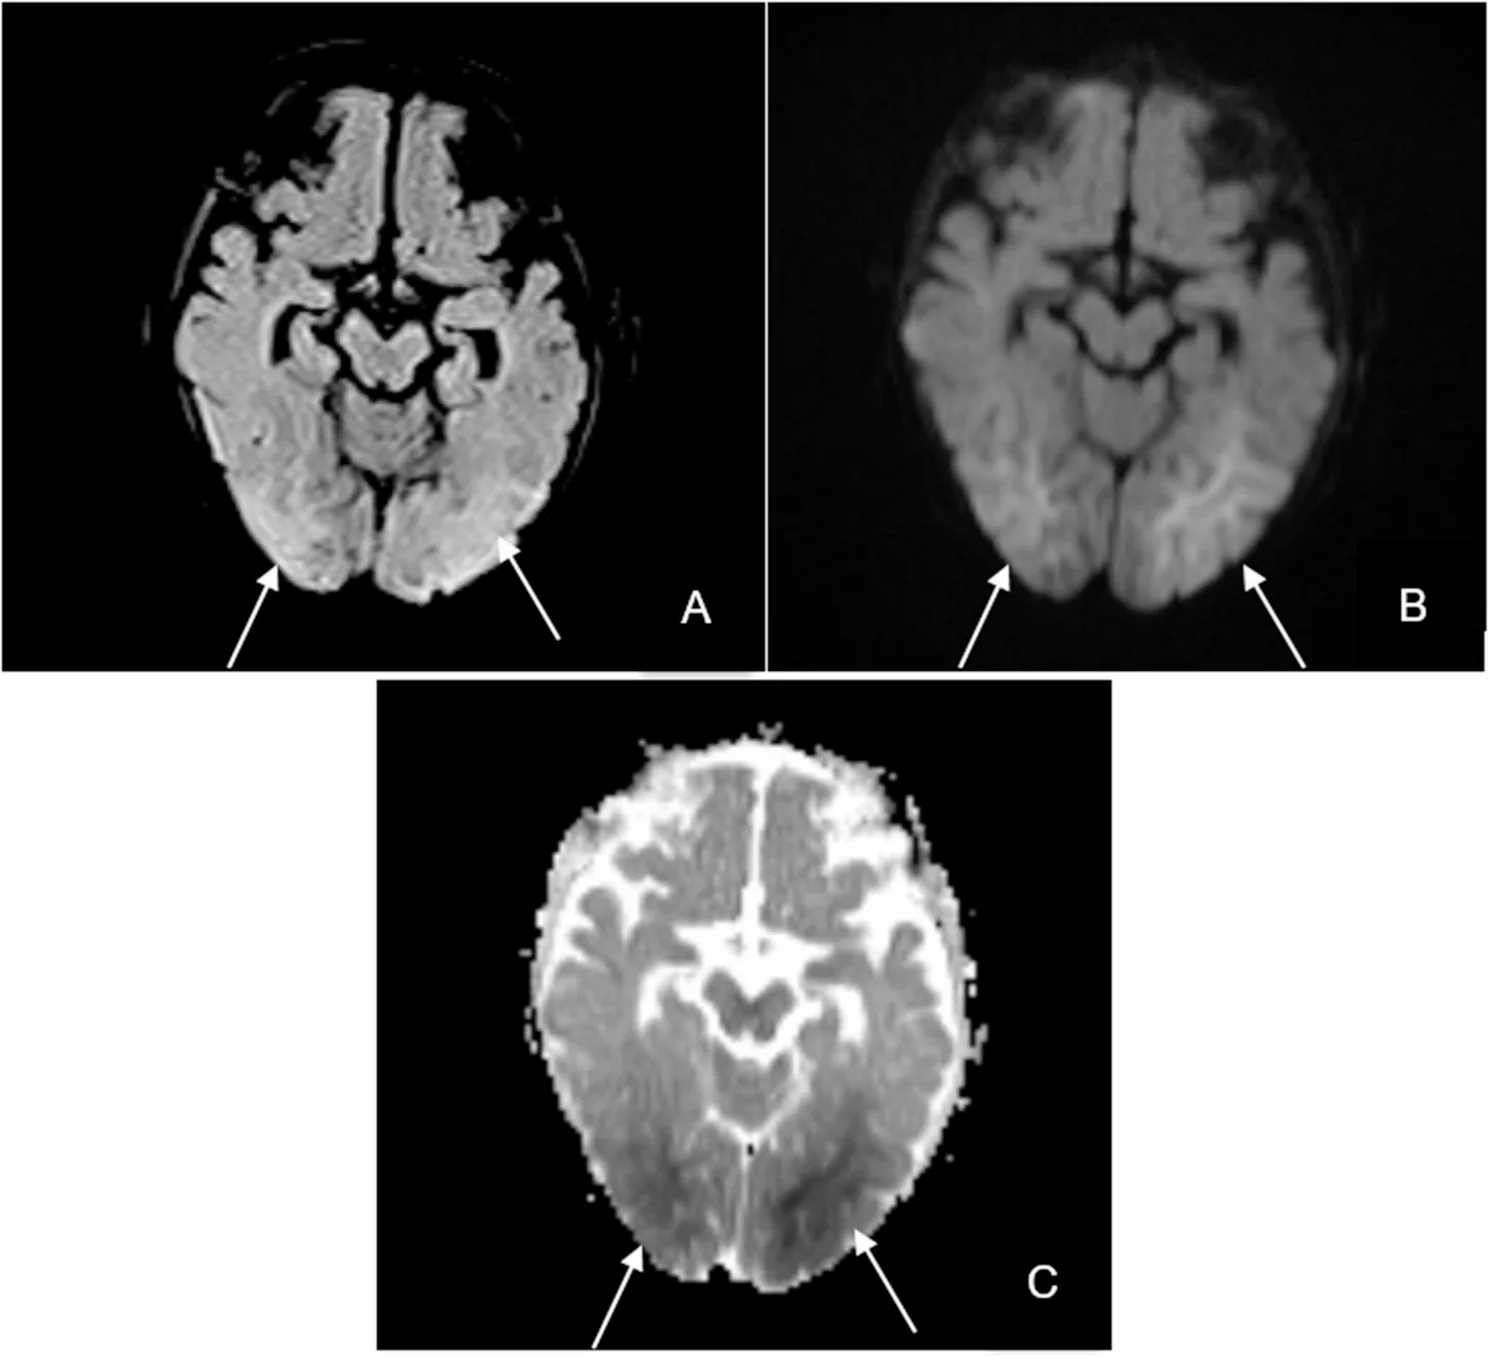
MRI identified a cortico-subcortical signal abnormality in the occipital lobes (thin arrows), bilaterally distributed with close symmetry. The lesions appear hyperintense on FLAIR (A) and diffusion-weighted imaging (B), with corresponding mildly reduced ADC signal on the ADC map (C). Overall, these findings are suggestive of PRES, predominantly vasogenic in pattern, with a possible superimposed cytotoxic component
